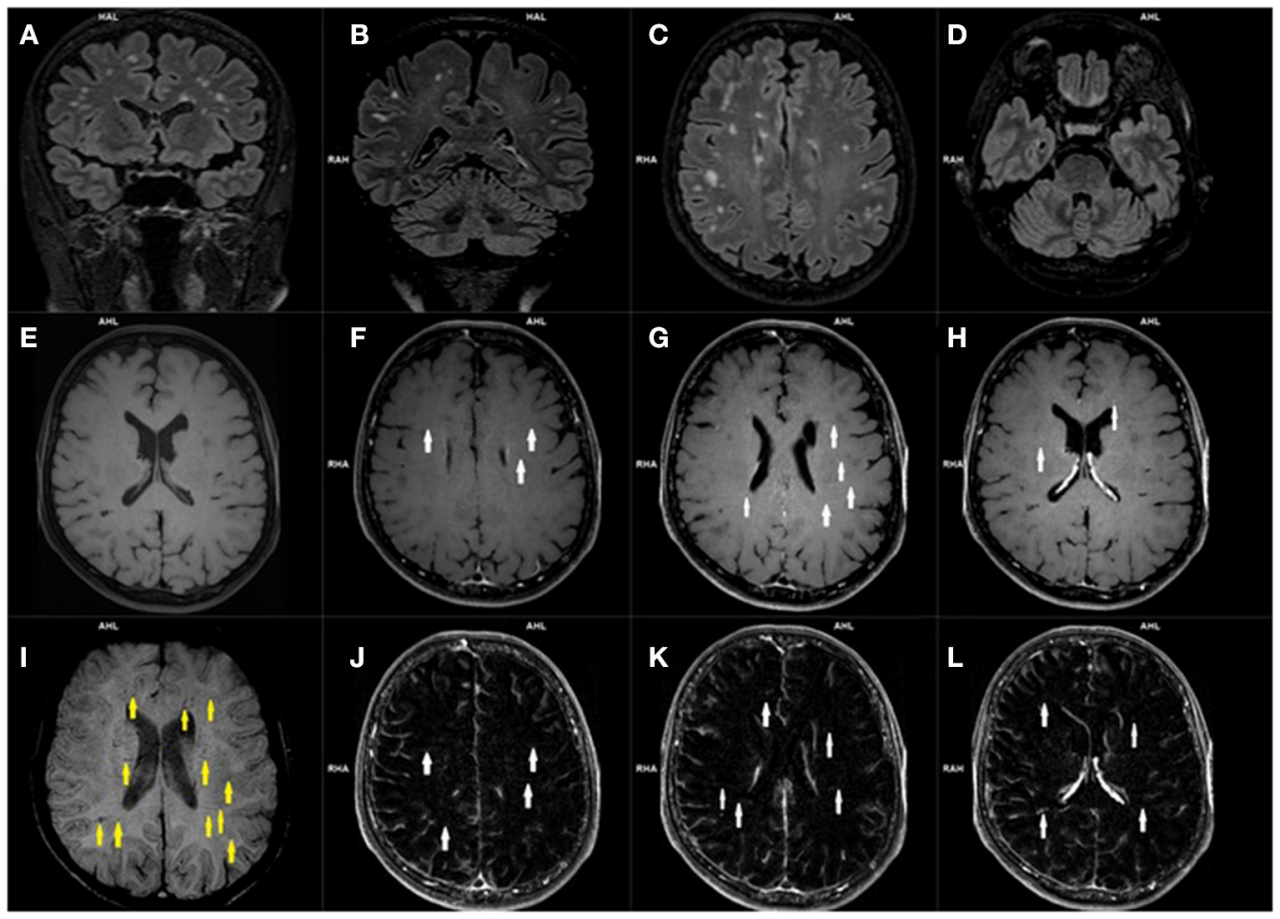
Magnetic resonance imaging of the brain of a patient with post COVID-19 symptoms. Coronal (A, B) and axial (C, D) 3D-FLAIR images demonstrate multiple hyperintensities located within subcortical and deep white matter. Axial precontrast T1-weighted image (E), and contrast enhanced axial T1-weighted images (E–H) with axial T1-weighted subtraction maps (J–L) show subtle parenchymal enhancement along the course of deep located parenchymal veins (*white arrows*). Corresponding SWI image (I) demonstrates engorged some deep medullary veins (*yellow arrows*).
